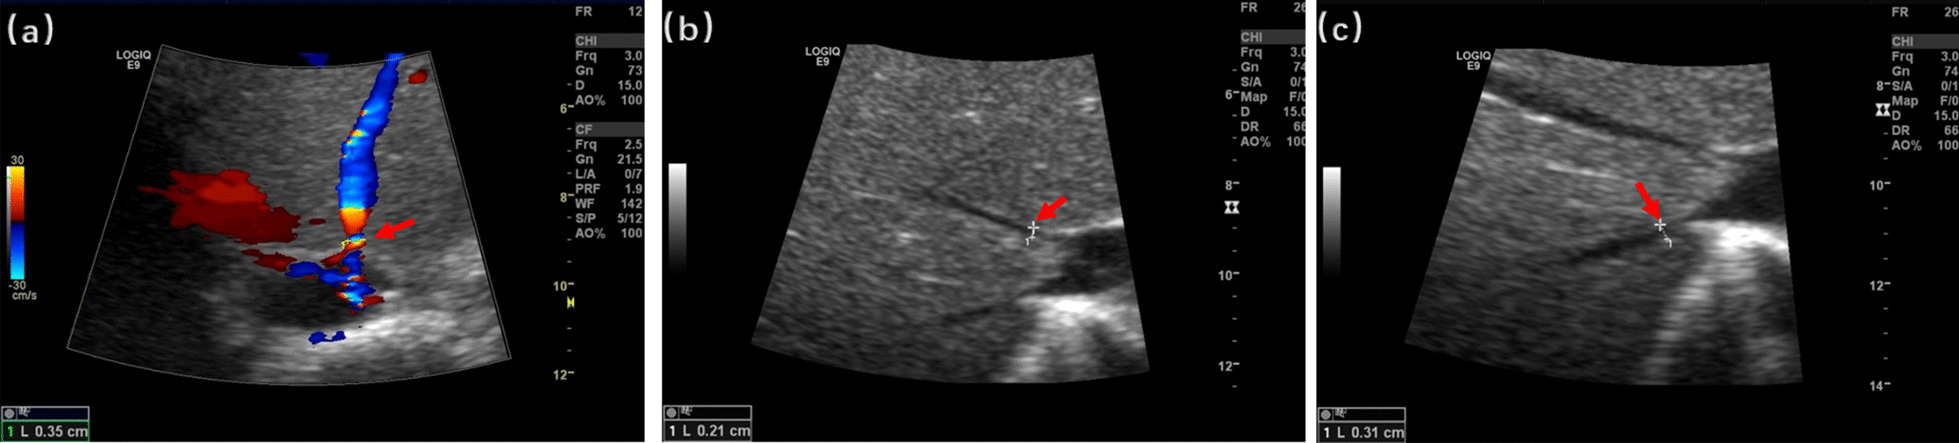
Ultrasound showed that the inner diameter on the left, middle, and right hepatic vein joining the inferior vena cava was 3.5 mm (a), 2.1 mm (b), and 3.1 mm (c)
